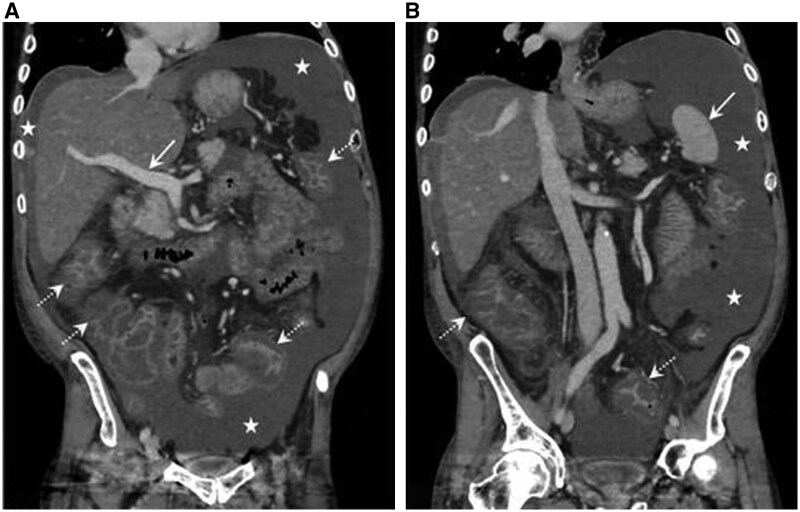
Coronal post-contrast computed tomography images at the time of admission reveal a large amount of ascites (asterisks). Recanalization of the umbilical vein (a collateral, secondary to portal hypertension) was clearly demonstrated (not shown). The liver is normal in size and contour, showing diffuse fat infiltration. The main portal vein is well visualized (arrow in A), with no evidence of portal or hepatic veins thrombosis. No splenomegaly is noted (arrow in B). Acute pancolitis is evident, as demonstrated by diffuse wall thickening of the large bowel loops (dashed arrows).
